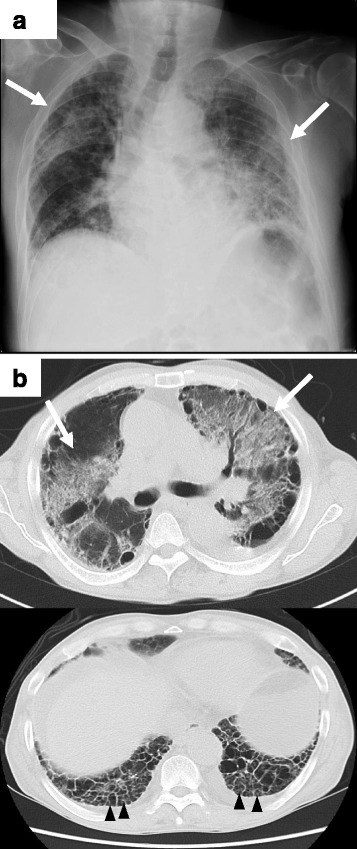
Findings of chest radiograph and computed tomography at admission. a
